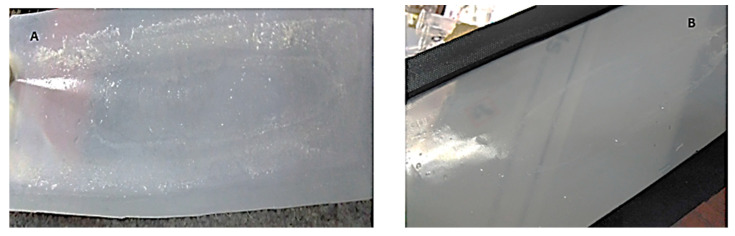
CTA membrane after experiment; (A): Before manual scrubbing; (B): after manual scrubbing.
